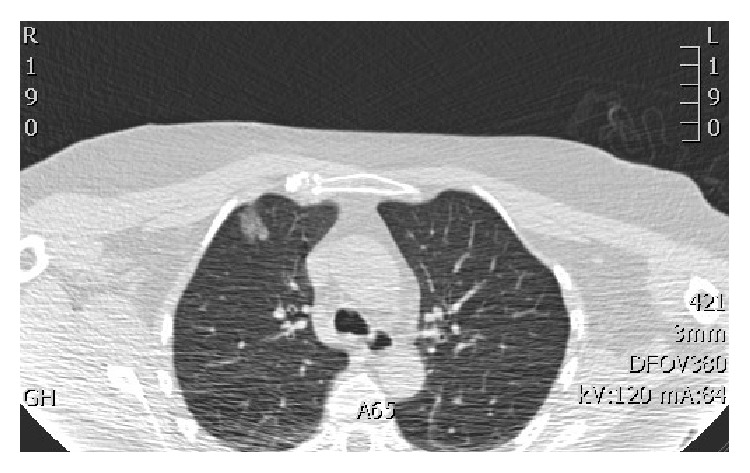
Image capture from CT fluoroscopy: the needle has been retracted and removed. The high-density methylene blue/blood track to the pleural surface can be identified.
